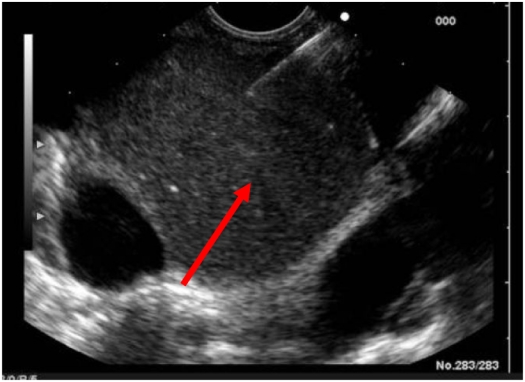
Endoscopic transoesophageal ultrasound guided fine needle aspiration (arrow - hyperechoic particles within cyst)
